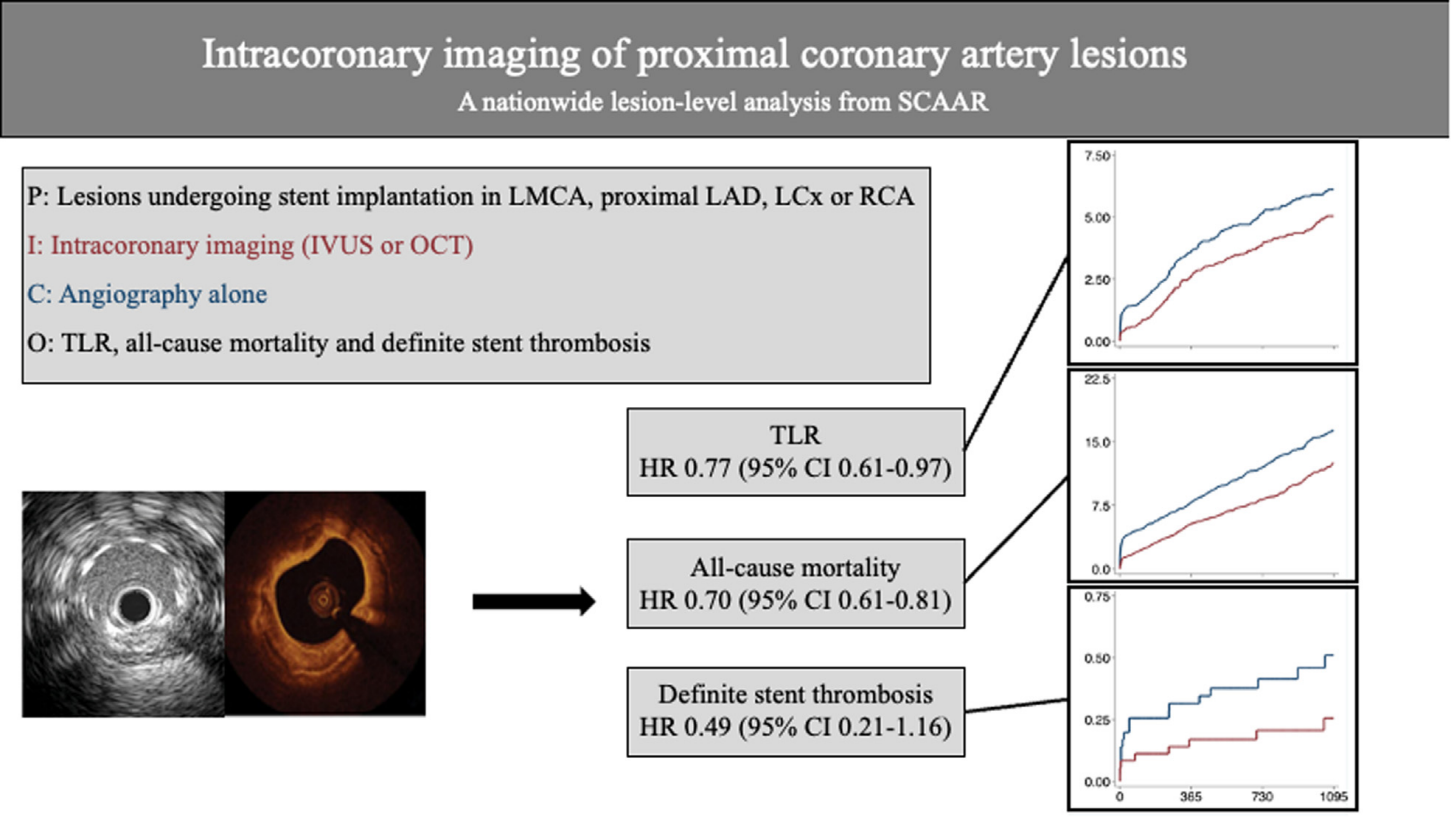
HR, hazard ratio; IVUS, intravascular ultrasound; LAD, left anterior descending; LCx, left circumflex artery; LMCA, left main coronary artery; OCT, optical coherence tomography; PCI, percutaneous coronary intervention; RCA, right coronary artery; SCAAR, Swedish Coronary Angiography and Angioplasty Registry; TLR, target lesion revascularization.
